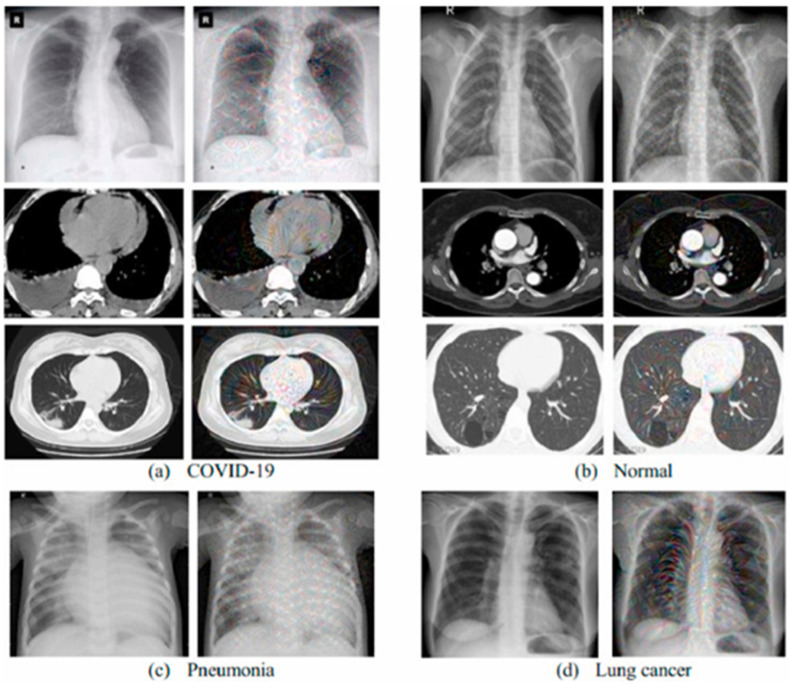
CT and X-ray Medical Images utilized for Diagnosing COVID-19 and distinguishing from other diseases (Ramdani et al., 2021) [17]. (a,b) shows CT images of COVID-19 of normal patient, while (c,d) shows CXR images of Pneumonia and lung cancer.
